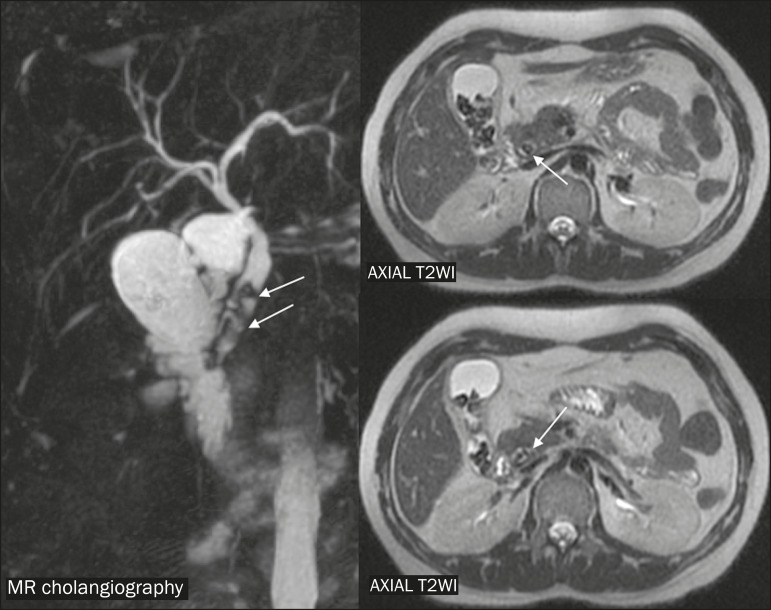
Magnetic resonance cholangiography and axial T2-weighted image (axial T2WI) from an MRI scan, showing choledocholithiasis, highlighting impacted calculi in the distal common bile duct (arrows), with mild dilatation of the upstream biliary tract.
